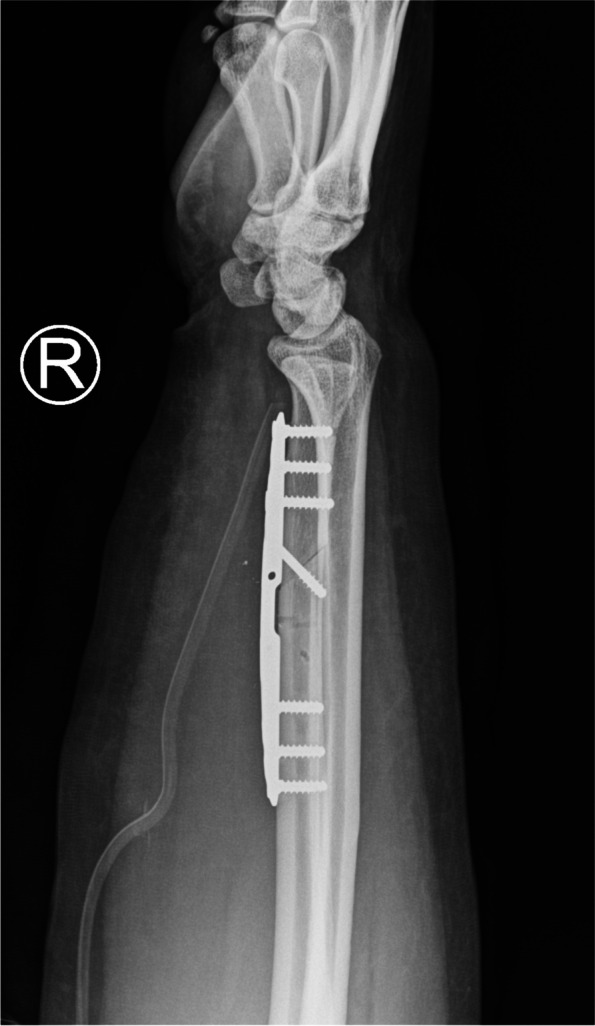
Postoperative radiograph of diaphyseal oblique USO
